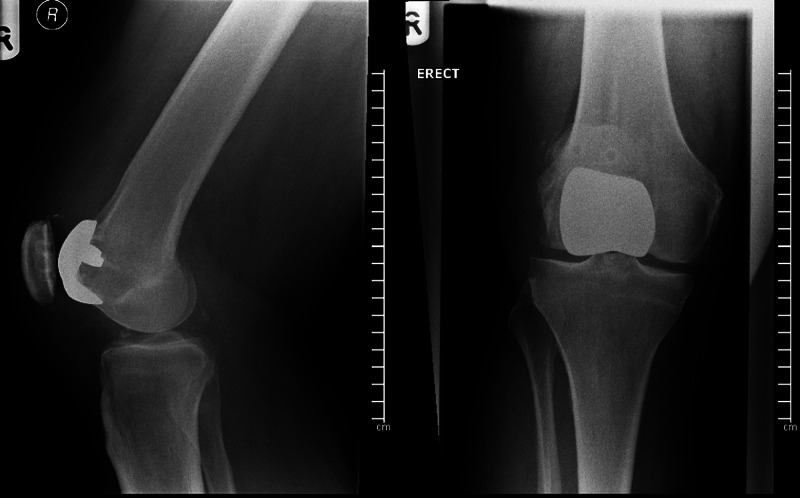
Post-operative radiographs of right knee following primary femoro patella vialla arthroplasty. Antero-posterior (right) and lateral (left) non-weight bearing radiographs of the right knee following primary patellofemoral arthroplasty. The femoral FPV component is well positioned on the trochlea. The patella button is correctly positioned in the patella and congruent with the femoral implant component.
